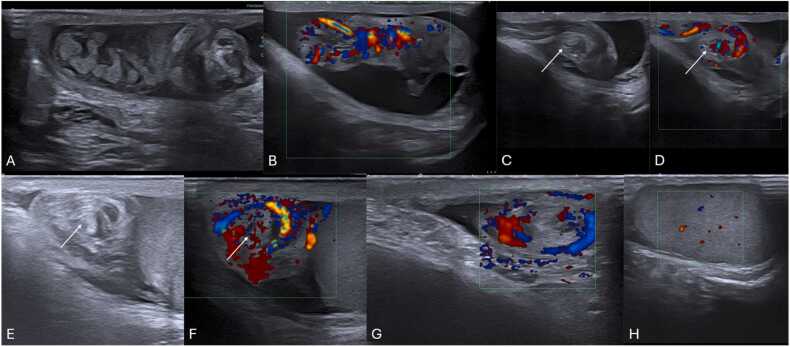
(A–H) Coupled testicular b-mode and color Doppler US images in the sagittal and transverse planes in different adolescent male patients with surgically proven testicular torsions. (A–F) Different examples of the twisted spermatic cord (“Whirlpool sign”), a direct sign of torsion. Note the twisting of the cord, particularly at the center of the twist (arrows). (G and H) Scrotal US images in a 15-year-old male, depicting the presence of a twisted spermatic cord (“Whirlpool sign”) with preserved testicular blood flow. The radiological diagnosis was suggested based on identification of the twisted spermatic cord. This case is an important reminder for the sonographer to systematically search for this sign in suspected testicular torsions.
